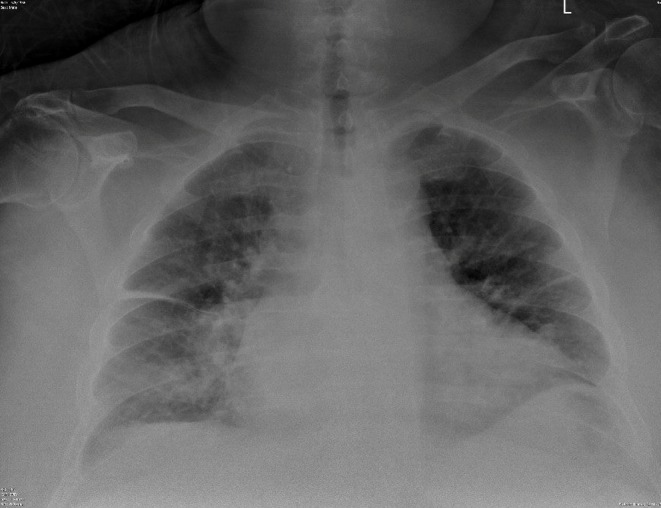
Chest radiograph showed cardiomegaly and findings suggestive of mild acute heart failure, with bilateral pleural effusions (right > left) and mild atelectasis.
